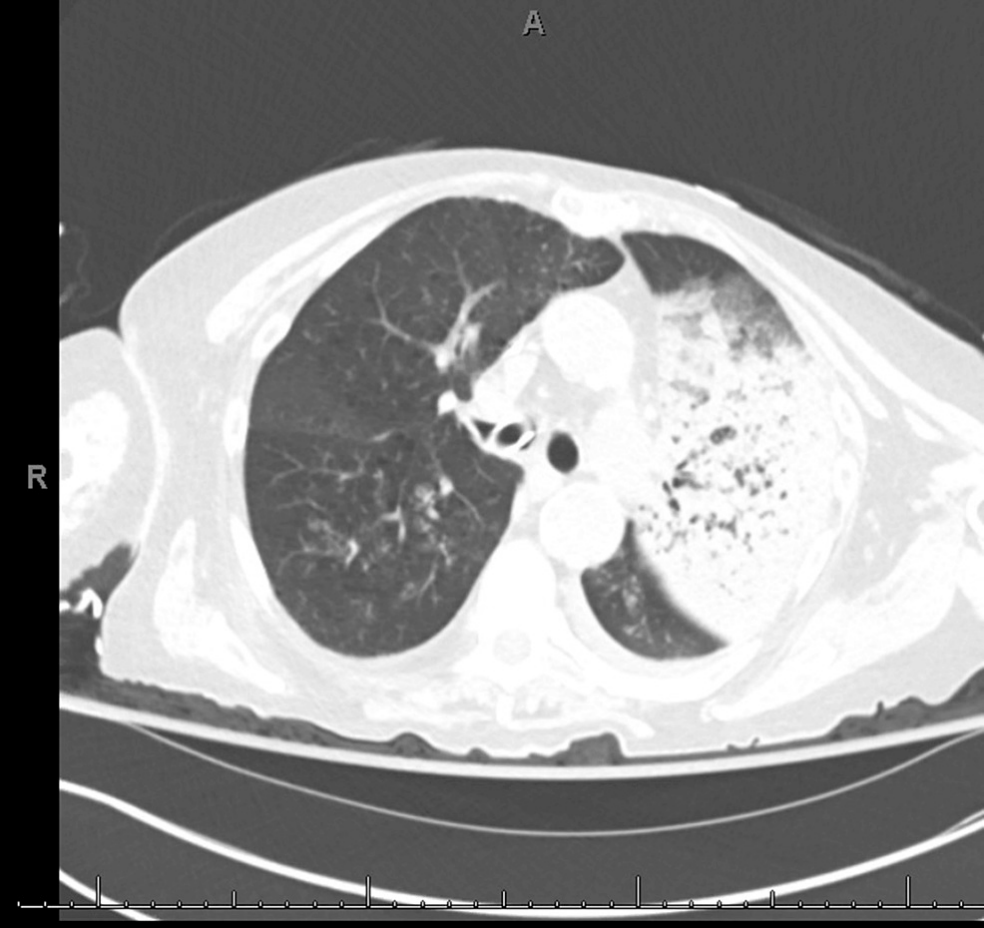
Non-contrast CT scan showing hemorrhage within left lung field (Patient Two).
